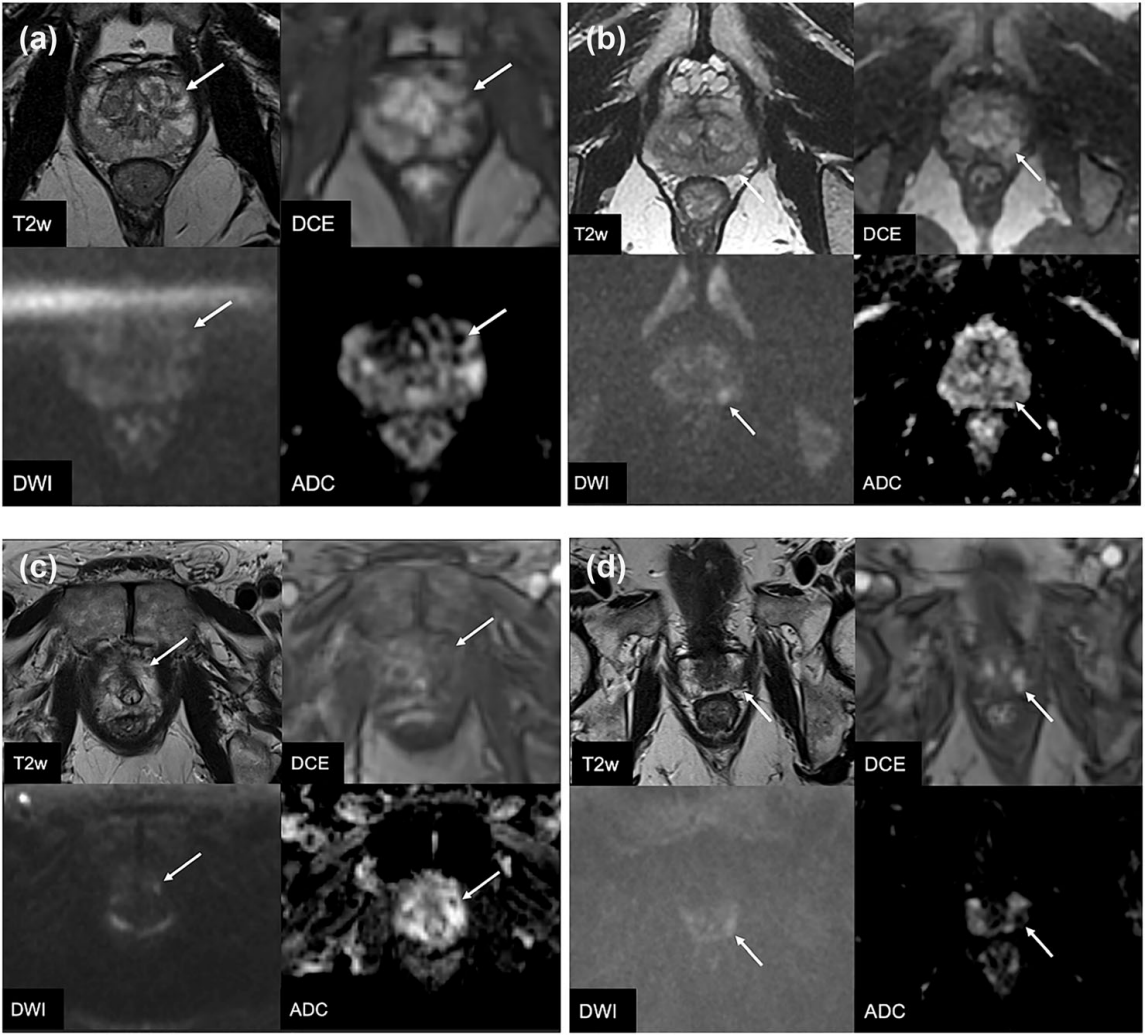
Representative MRI images of (A) PIRADS 3− with negative biopsy, (B) PIRADS 3+ with GG2 PCa on biopsy, (C) PIRADS 4− with negative biopsy, and (D) PIRADS 4+ with GG3 PCa on biopsy.
